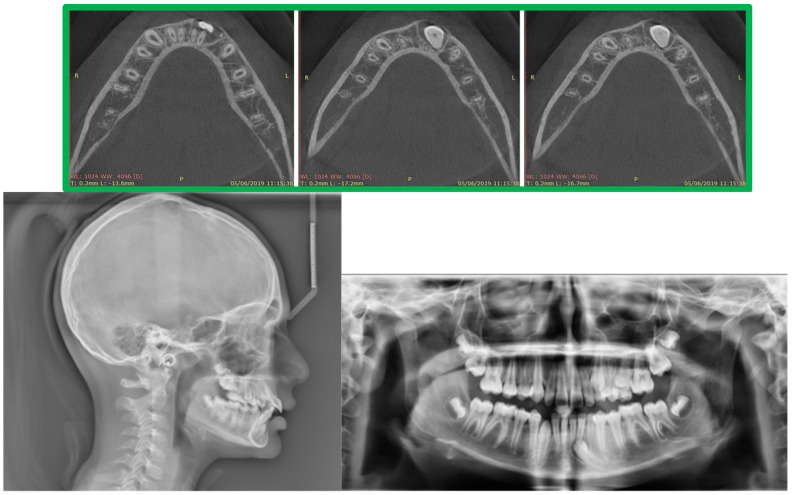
CBCT, cephalometric radiographs, and pretreatment panoramic.
